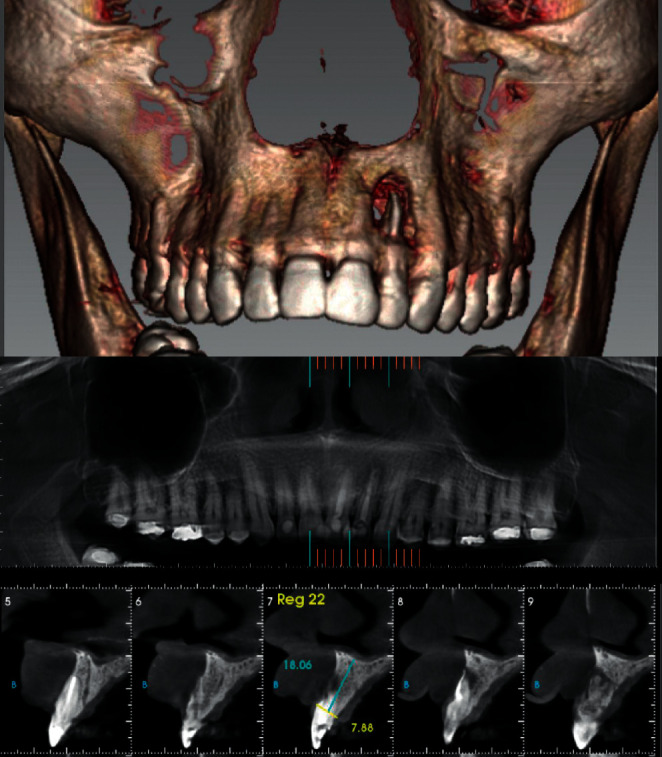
Initial CBCT images of the periapical lesion of tooth #22.
